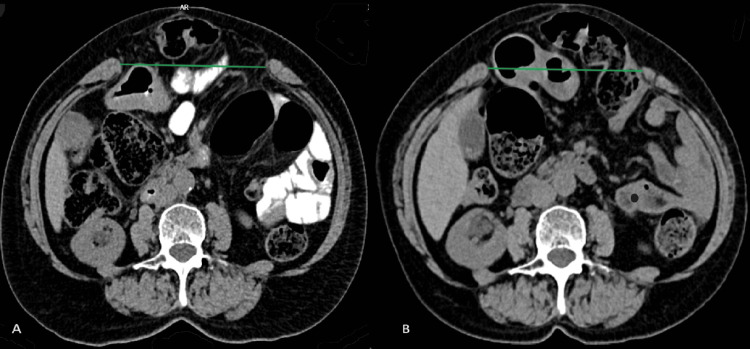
Transversal abdominal CT images showing incisional hernia before and four weeks after botulinum toxin A infiltration A: Before preoperative botulinum toxin A infiltration; B: Four weeks after preoperative botulinum toxin A infiltration The green lines represent the hernia width.
